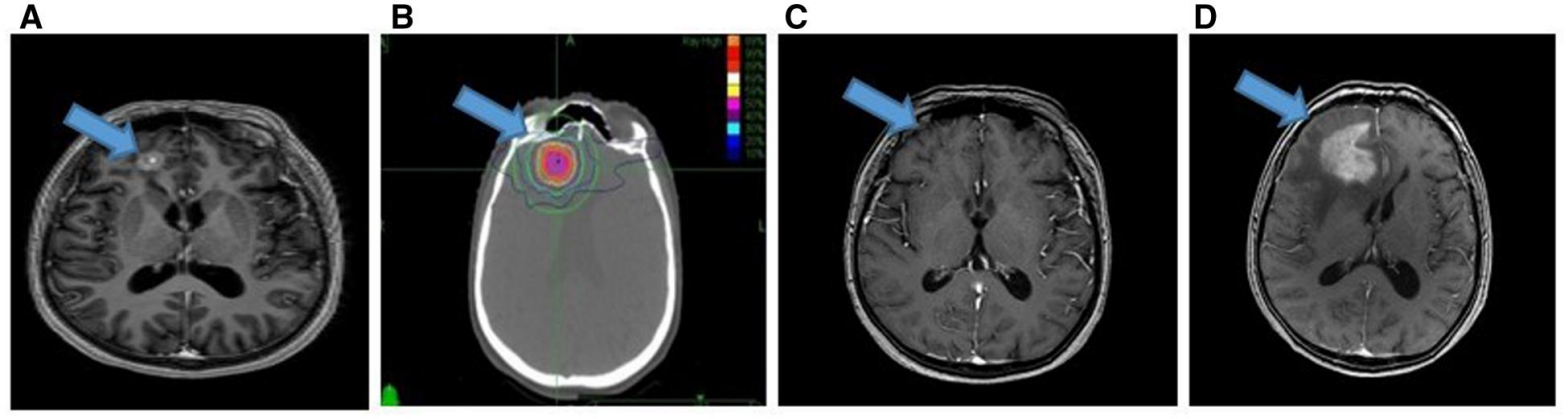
(A) T1W brain MRI sequence shows heterogeneous contrast enhancement in the right frontal region, consistent with a 12-mm diameter metastasis. (B) CT image of SRT planning. (C) MRI T1A sequence image 6 months after treatment; complete response. (D) MRI image at the 12th month after treatment. Pathologically confirmed MRI T1A sequence of radionecrosis image with peripheral oedema in the right frontal region, contrast enhancement in the middle and soap bubble appearance.
